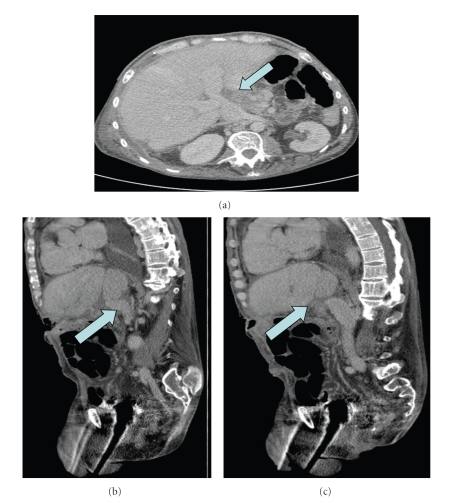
CT venogram showing cavoportal hemitransposition with the donor liver anastomosed to the recipient vena in the piggyback fashion (see arrow).
